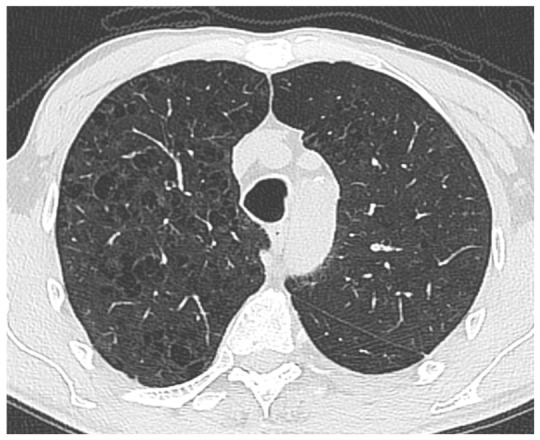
Thoracic computed tomography image demonstrating multiple small, irregularly shaped areas of low attenuation diffusely distributed in the upper lobes of both lungs. These findings are suggestive of centrilobular emphysema. The absence of a peripheral predominance and the localization of lesions adjacent to the central bronchioles are consistent with a typical centrilobular pattern.
